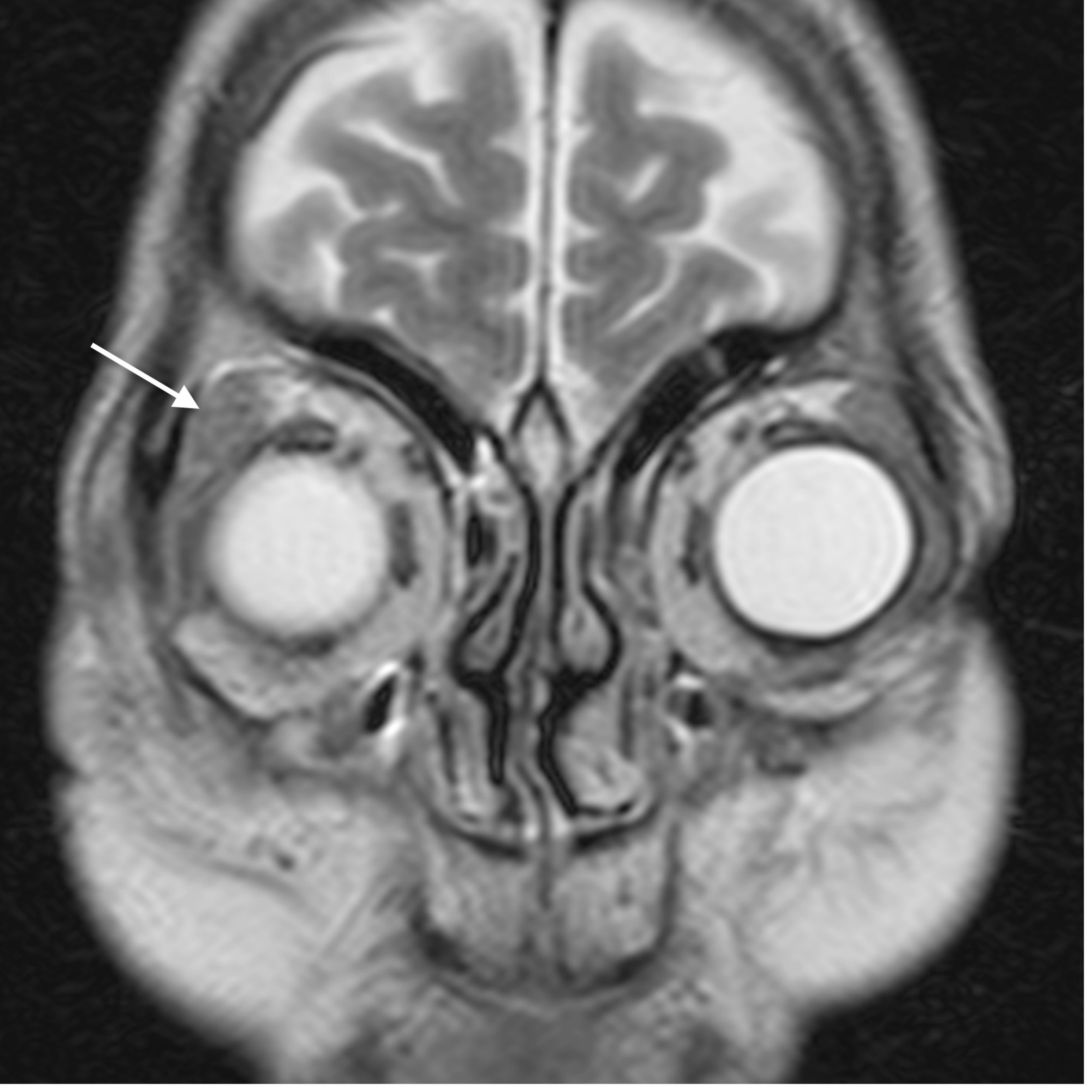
Dacryoadenitis: coronal T2WI shows bulky right lacrimal gland with slight heterogeneous signal (arrow) T2WI - T2-weighted image
